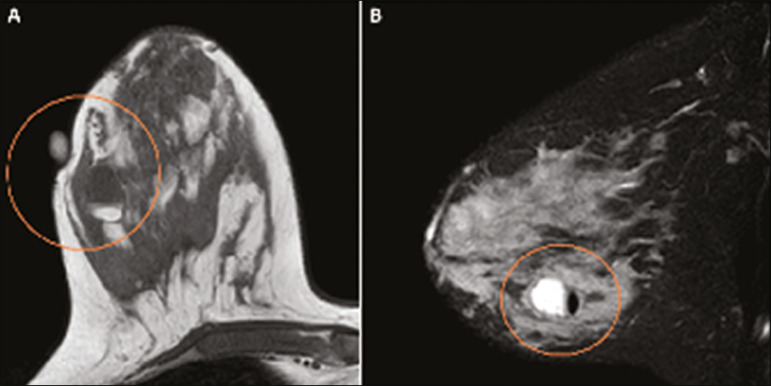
T1 (A) and T2 (B) weighted MRI images showing cyst with thin septa, heterogeneous content, and fat-fluid level, which is compatible with fat content/galactocele.
